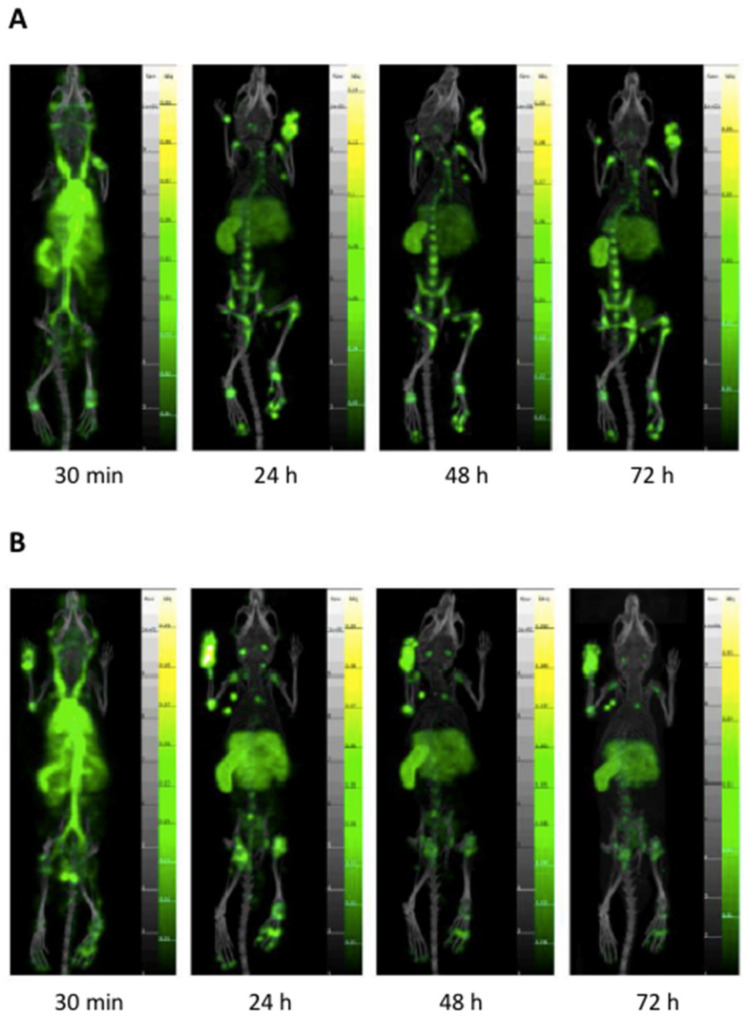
Pharmacokinetics of ^111^In-labelled liposomes encapsulating methotrexate (MTX) assessed by nuclear imaging Single Photon Emitted Computed Tomography (SPECT-CT). (A) Non-targeted liposomes (L-MTX). (B) Folate-targeted liposomes (FL-MTX). Please note at 30 min the early targeting of inflamed joints and the strong signal from vessels. Targeting in inflamed joints remains very stable over time in inflamed joints.
